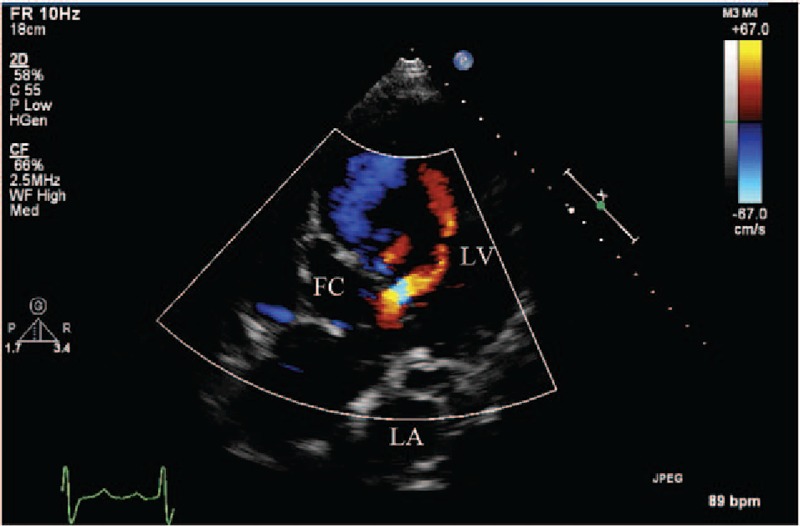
(Patient #11) Transthoracic echocardiogram (apical 5 chamber view with color) showing 2 flow jet communications from interventricular septal dissecting cavity to the left ventricular cavity. FC = false cavity, LA = left atrium, LV = left ventricle.
